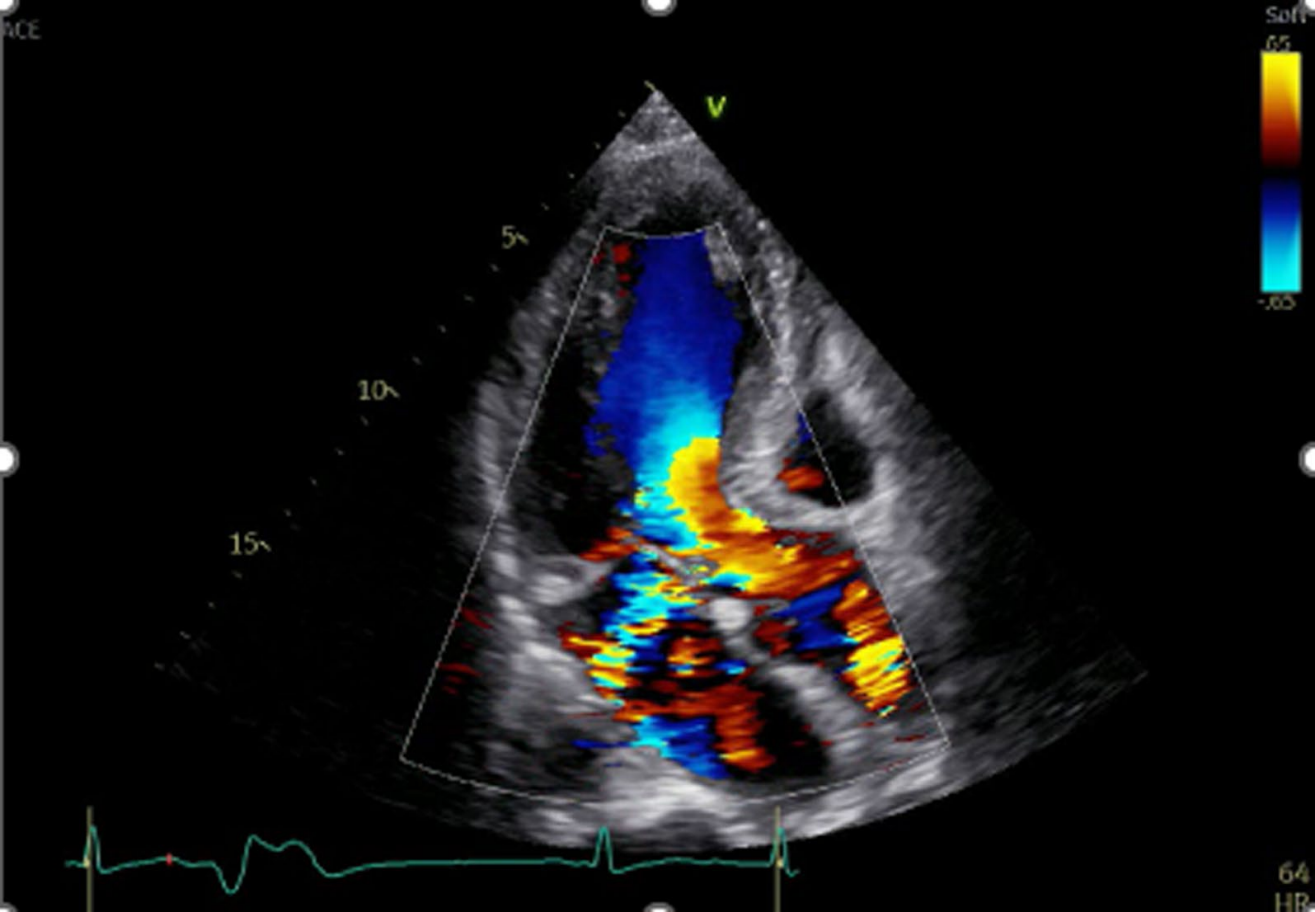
Transthoracic echocardiography revealed the pseudoaneurysm and a backflow into the left atrium through the midline of the anterior mitral leaf (AML). There was moderate aortic regurgitation (AR) and the direction of the AR jet faced to the pseudoaneurysm
